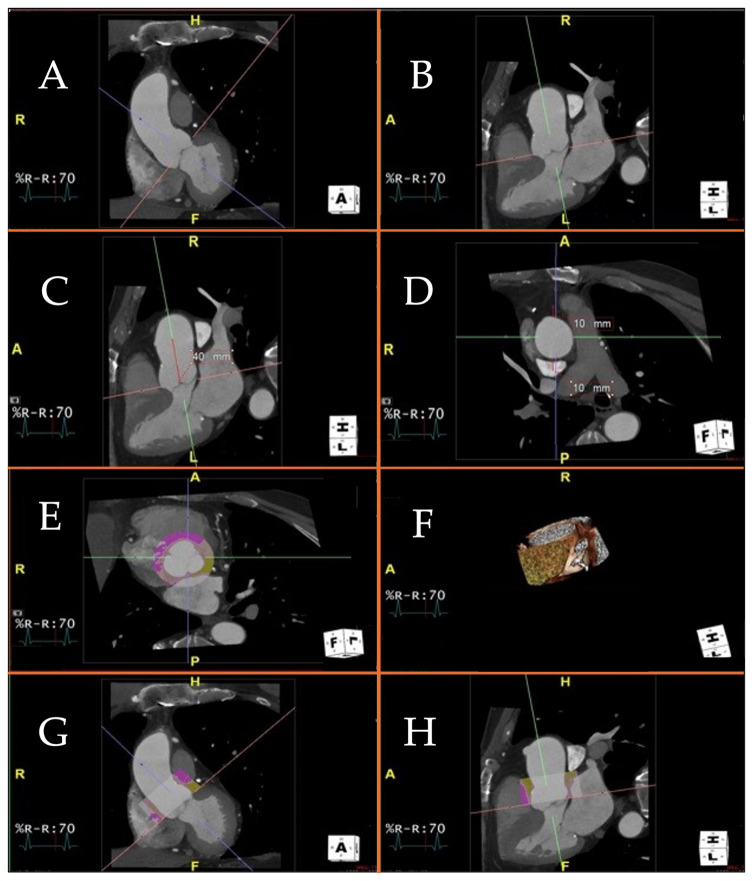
Figure 1 displays a visualization of the cardiac planes and ascending aorta using a 3D platform and the MultiPlanar Reconstruction (MPR) application. Specifically, the MPR mode enables accurate positioning of the cardiac viewing planes for the calculation of perivascular adipose tissue attenuation. Technical steps required to measure perivascular adipose tissue attenuation of the proximal ascending thoracic aorta on chest computed tomography angiography. (A–C): different projections of the ascending aorta and aortic valve. You can see the projections in two (A) and three (B,C) chambers, respectively; (D): axial visualization of the ascending aorta, at the pulmonary trunk; (E): Axial visualization of the aortic valve, at the three cusps, in diastolic phase. The colored circular section indicates the application of the software to measure perivascular adipose tissue attenuation of the proximal ascending thoracic aorta and aortic bulb; (F): Visualization volume rendering of the aortic bulb, at the origin of the coronary arteries; (G): Two-chamber visualization of the ascending aorta. Colored section indicates software application to measure perivascular adipose tissue attenuation of the proximal ascending thoracic aorta and aortic bulb; (H): Three-chamber visualization of the ascending aorta. Colored section indicates software application to measure perivascular adipose tissue attenuation of the proximal ascending thoracic aorta and aortic bulb.
